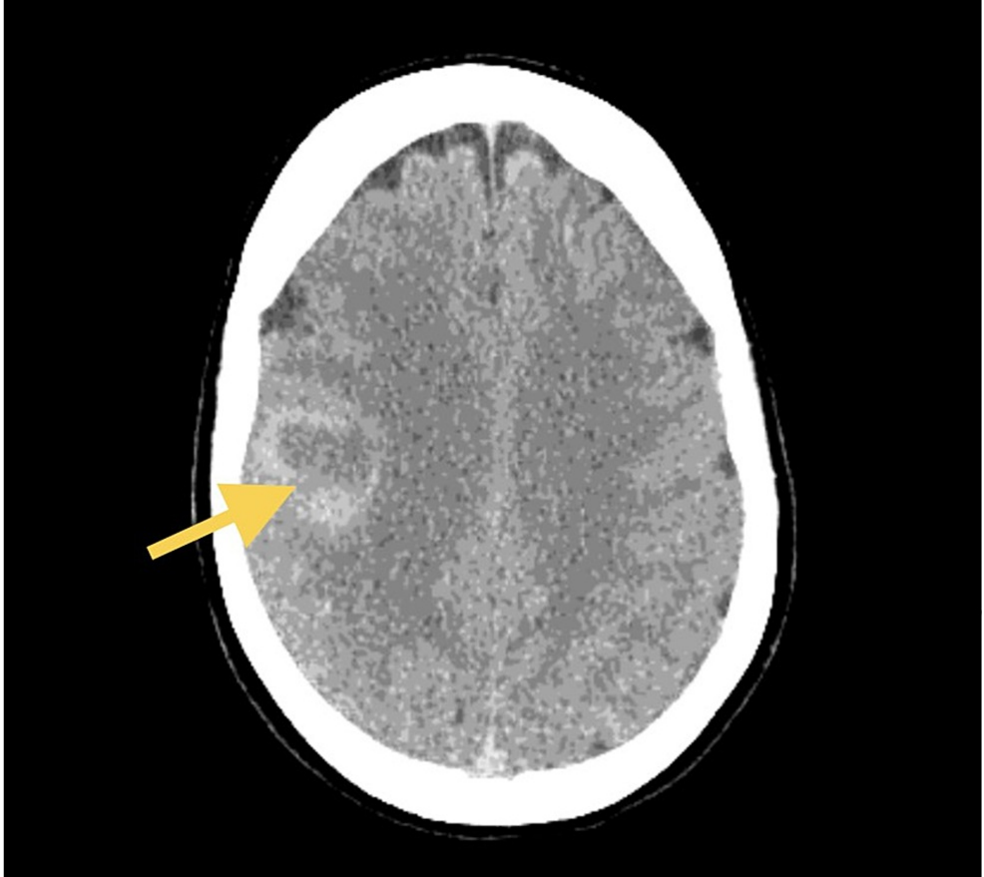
Computed tomography (CT) head with the yellow arrow showing 3.0 X 2.9 cm mass in the right cerebral hemisphere near the gray-white junction.
